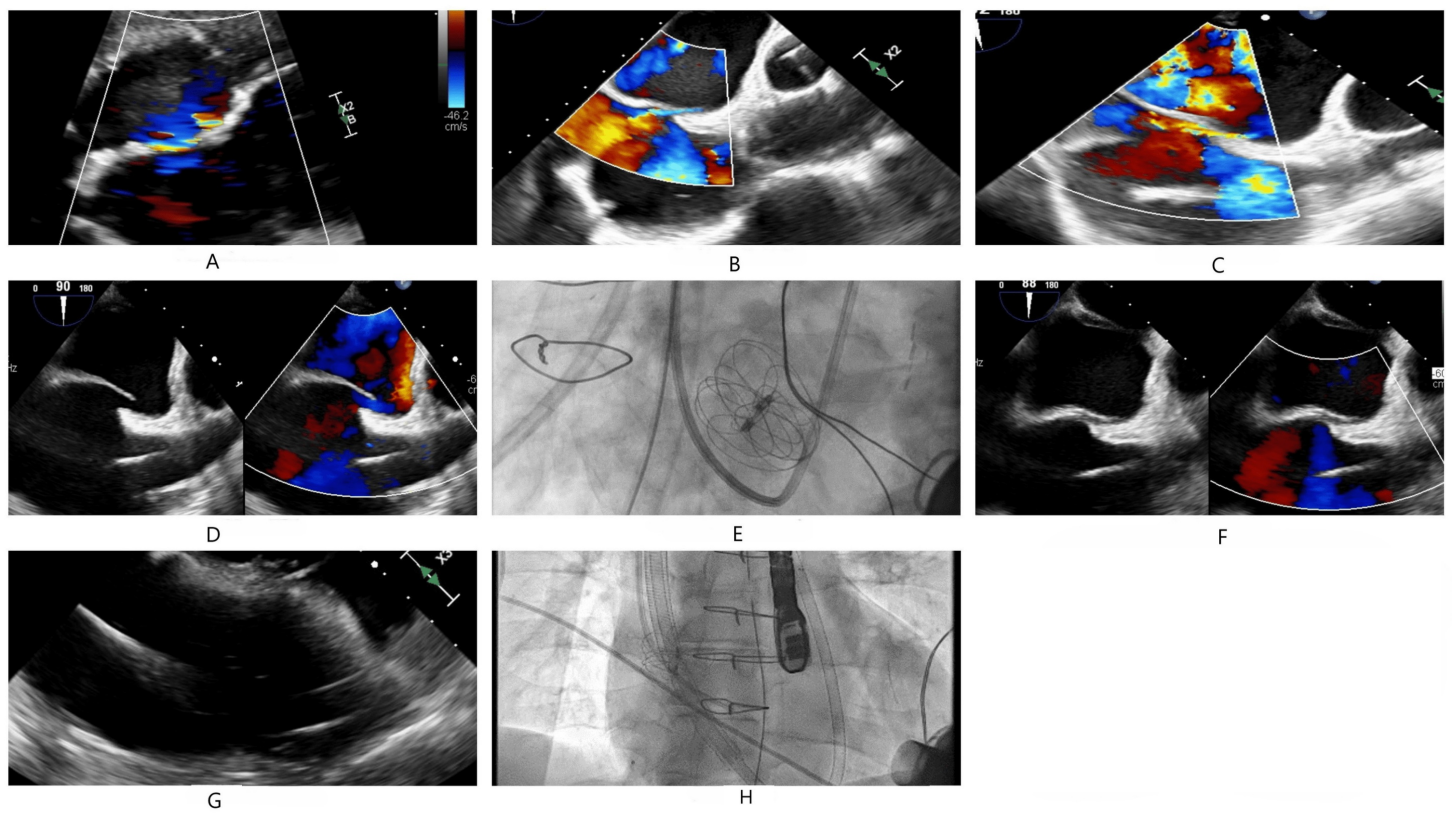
Evolution of the patent foramen ovale shunt with serial interventions A) Pre-intervention TEE demonstrating a left-to-right shunt; B) Pre-intervention TEE demonstrating a left-to-right shunt; C) Post-LVAD TEE demonstrating a reversed right-to-left shunt; D) PFO pre-closure; E) Amplatzer device placement, fluoroscopic view; F) Closed PFO; G) Right ventricular dysfunction; H) Protek Duo right ventricular mechanical support LVAD: left ventricular assist device; PFO: patent foramen ovale; TEE: transesophageal echocardiogram
